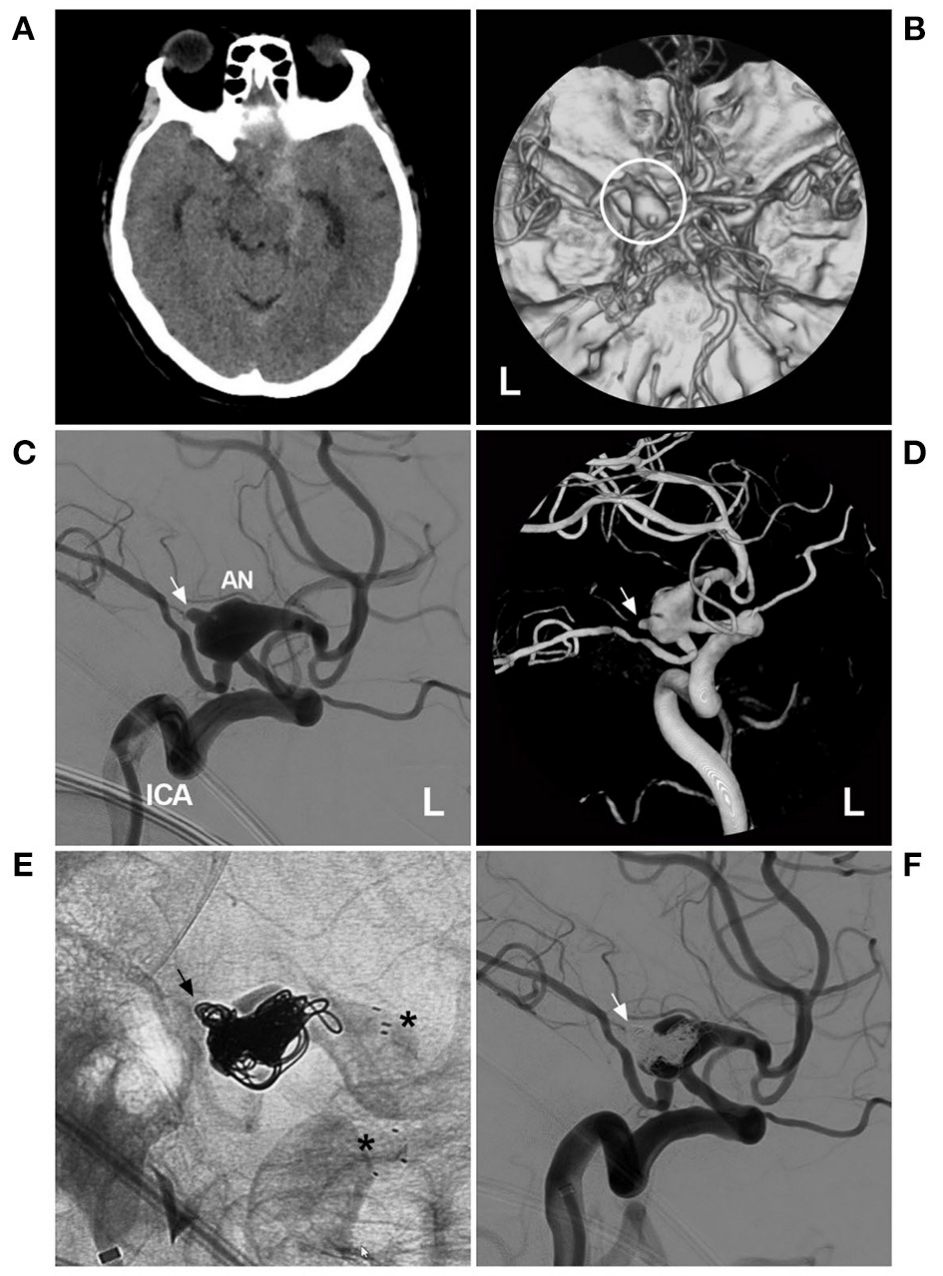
Targeted coiling for a circumferential AN of the PcomA. (A) CT showed right subarachnoid hemorrhage around the brainstem and in the Sylvian fissure. (B) CTA showed a large AN of the left PcomA (circle). (C,D) DSA (C) and three-dimensional DSA (D) revealed the circumferential AN of the left PcomA segment, with a ruptured bleb on the top (arrow). (E) X-ray film showed that the AN had been embolized with stent assistance (asterisks) and that the ruptured bleb had been filled by coils (arrow). (F) DSA indicated that the ruptured bleb had been satisfactorily treated by targeted embolization (arrow). AN, aneurysm; CT, computed tomography; CTA, computed tomography angiography; DSA, digital subtraction angiography; L, left; PcomA, posterior communicating artery.
